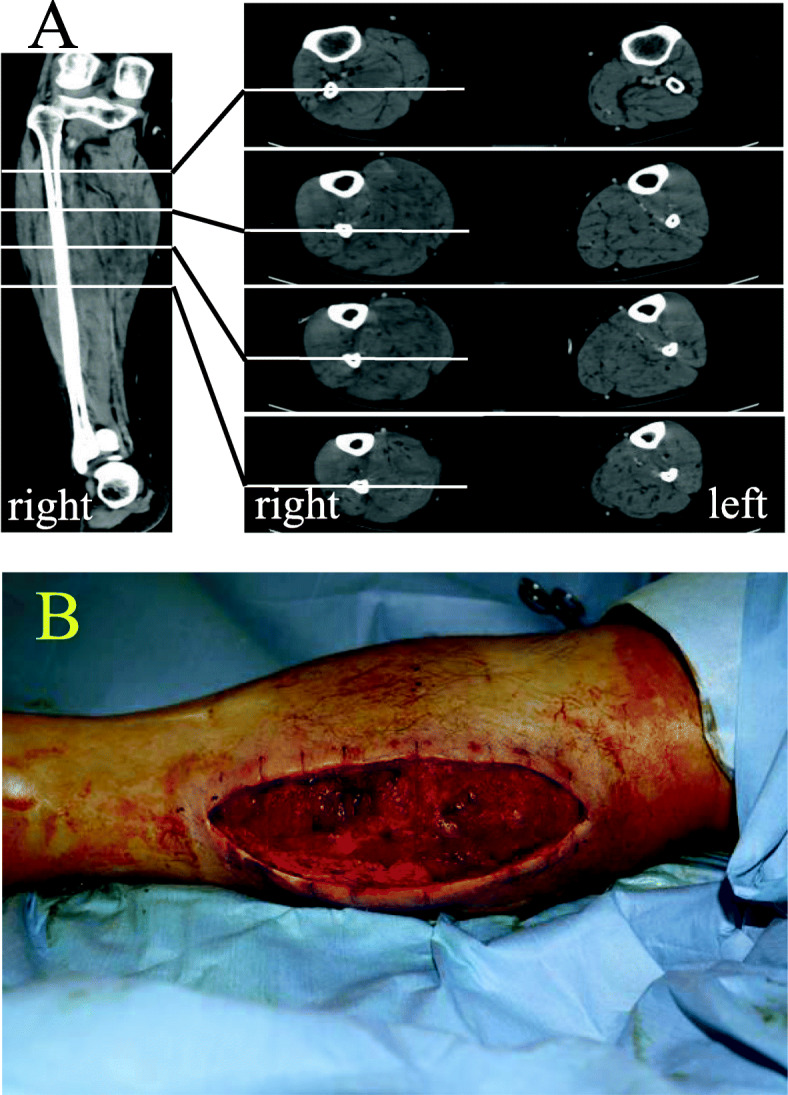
a Contrast-enhanced CT imaging of the lower limbs, and b the lateral aspect of the right lower limb after fasciotomies on both sides of the calf in the present case. Left and right panels of a present a coronal view of the right lower limb and axial views of bilateral lower limbs, respectively. Note that in a, the right lower limb shows an increase in size (i.e., swelling) and a decrease in contrast enhancement (i.e., hypoperfusion), compared with the left lower limb
